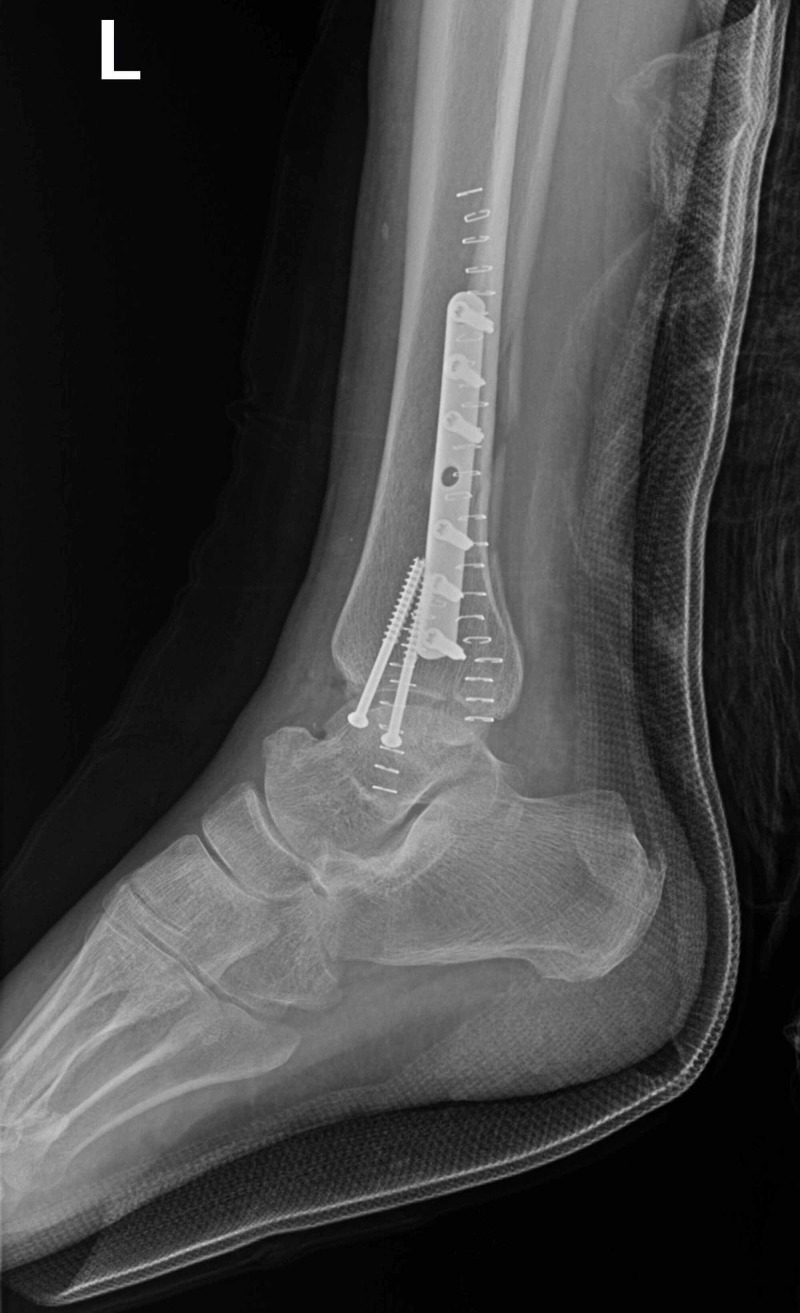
Post reduction lateral X-ray view visualizing complete repair and reduction of left ankle
